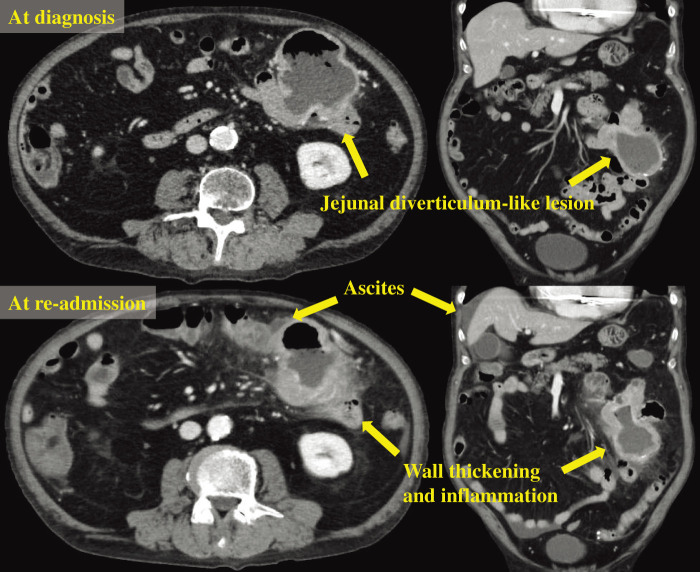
Preoperative CT imaging. A contrast-enhanced CT scan incidentally revealed a small intestinal mass. At readmission, CT demonstrated wall thickening around the jejunal diverticulum-like lesion and the development of ascites.
